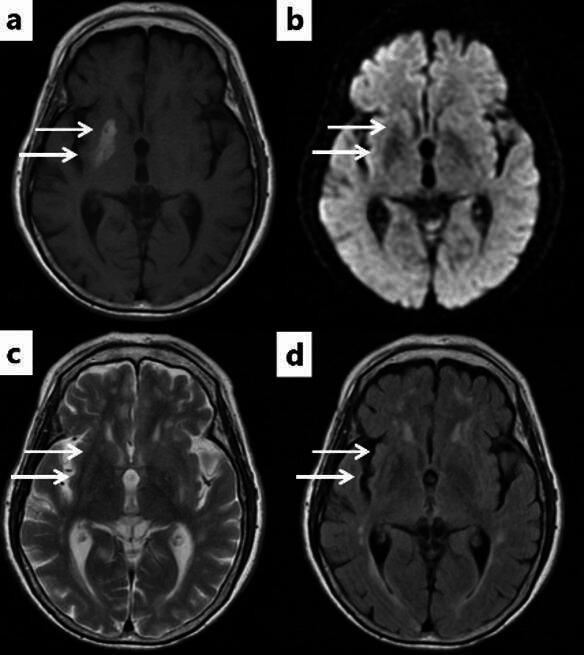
Non-ketotic hyperglycemic hemichorea. a T1WI, b DWI, c T2WI, d FLAIR. A woman in her 70s with type 2 DM presented with new-onset left arm and leg numbness. Axial T1WI (a) reveals marked unilateral hyperintensity in the basal ganglia (arrow) contralateral to the involuntary movements. Abbreviations: DM, diabetes mellitus; FLAIR, fluid-attenuated inversion recovery; DWI, diffusion-weighted imaging
